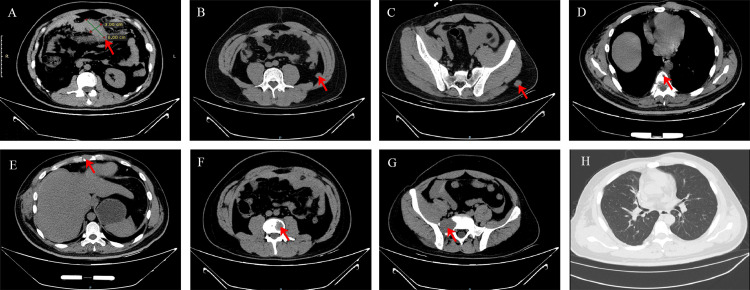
Non-contrast CT of the abdomen Non-contrast CT of the abdomen in axial planes reveals a soft tissue mass measuring approximately 6 cm × 3 cm in the greater omentum region (A, red arrow). Additional soft tissue masses are identified in the retroperitoneum (B, red arrow) and the left buttock (C, red arrow). New multiple osteolytic bone destructions are noted in the thoracic vertebrae (D, red arrow), sternum (E, red arrow), lumbar vertebrae (F, red arrow), and sacrum (G, red arrow). No significant lesions are observed in the lungs (H).
